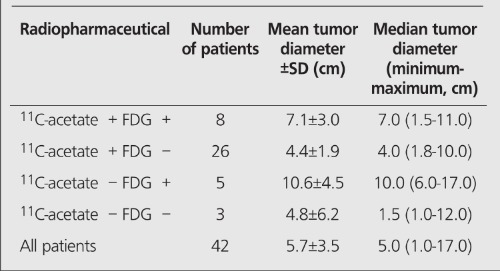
Largest tumor diameter in 42 evaluable patients with HCCvs. positive or negative finding at examinations with ^11^C-acetate andFDG, respectively
